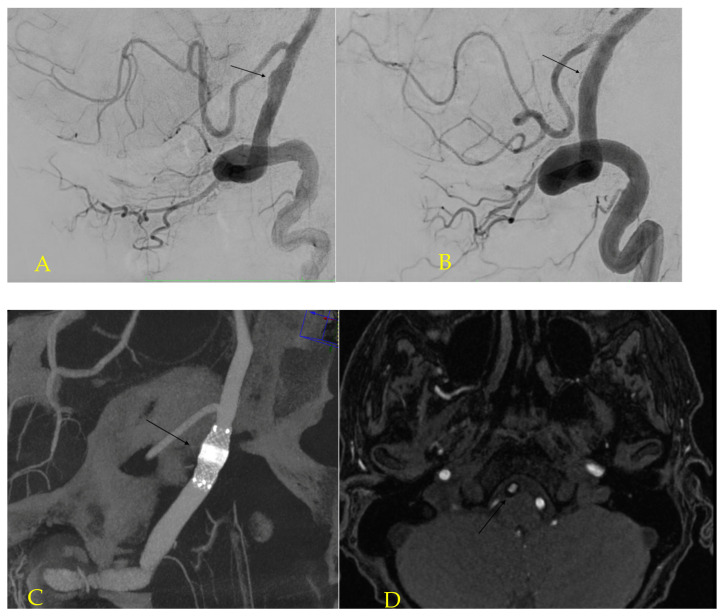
Pretreatment right vertebral lateral angiography (A) shows the blood blister-like aneurysm (arrow) below posterior inferior cerebellar artery (PICA). Postembolization angiography (B) reveals reduced filling of the aneurysm’s sac. Excellent apposition to the vessel wall and compacting the mesh around the aneurysm’s neck are visualized on intraprocedural contrast-enhanced flat panel detector CT (MIP reconstruction) (C). One year following the treatment, MRI revealed good positioning of the stent (arrow) and complete occlusion of the aneurysm (D).
